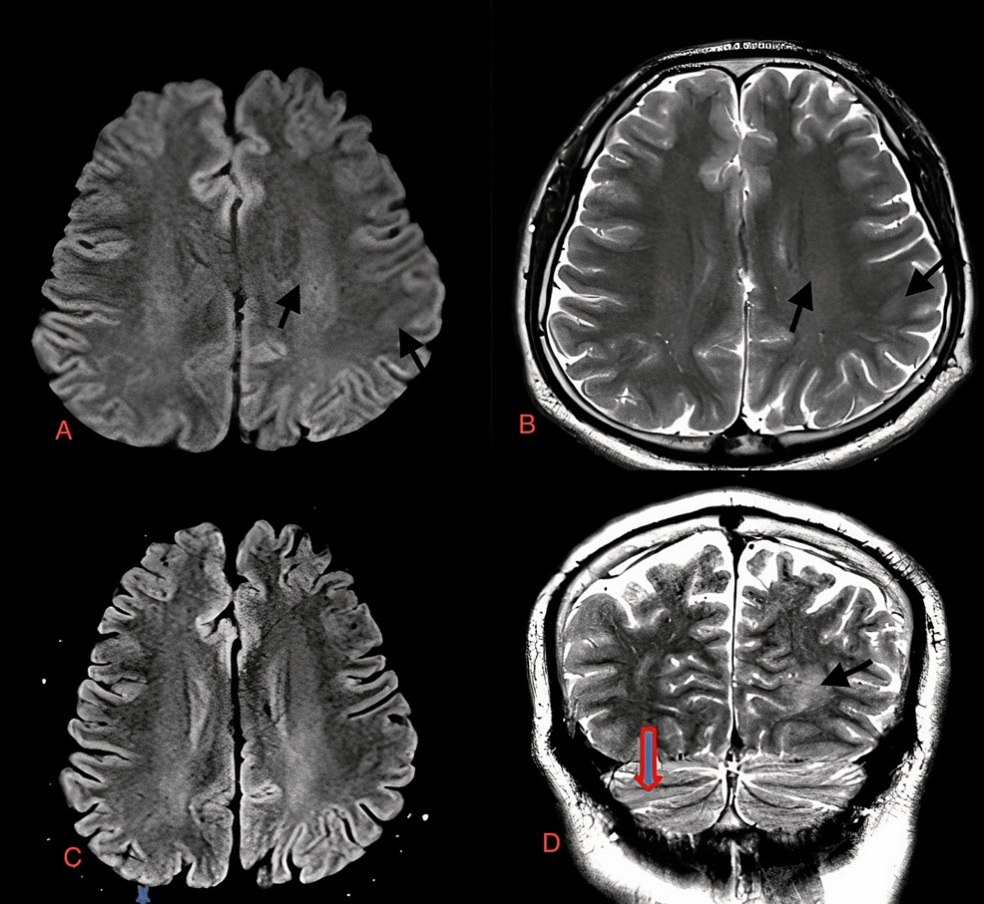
Case 2: Asymmetrical hyperintensities are seen in the deep and subcortical white matter of the left parietal lobe on DWI axial (A) and T2W axial (B) images in a 28-year-old male with dengue encephalitis. An area of cerebellar hyperintensity is present in the T2W image (D). No diffusion restriction is seen in the FLAIR image (C). T2W: T2-weighted image; DWI: diffusion-weighted image; FLAIR: fluid-attenuated inversion recovery sequence
